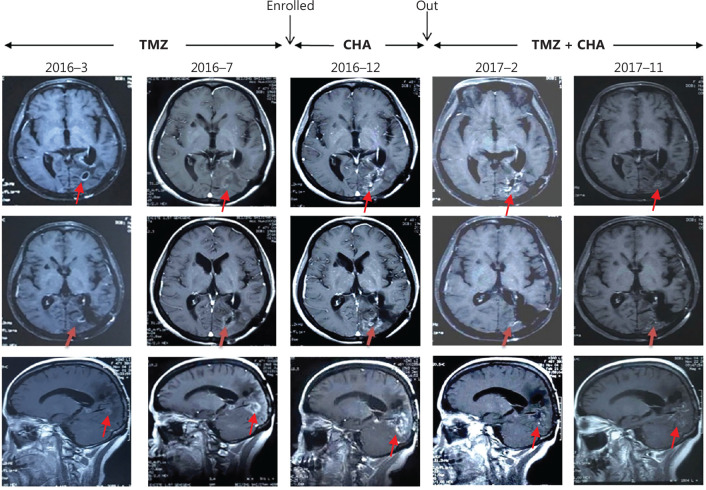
MRI images (arrows indicate tumors) obtained at baseline, 4 weeks of treatment, and follow-up of a representative patient (ID 0005) ww chlorogenic acid treatment. Red arrows indicate tumors.
